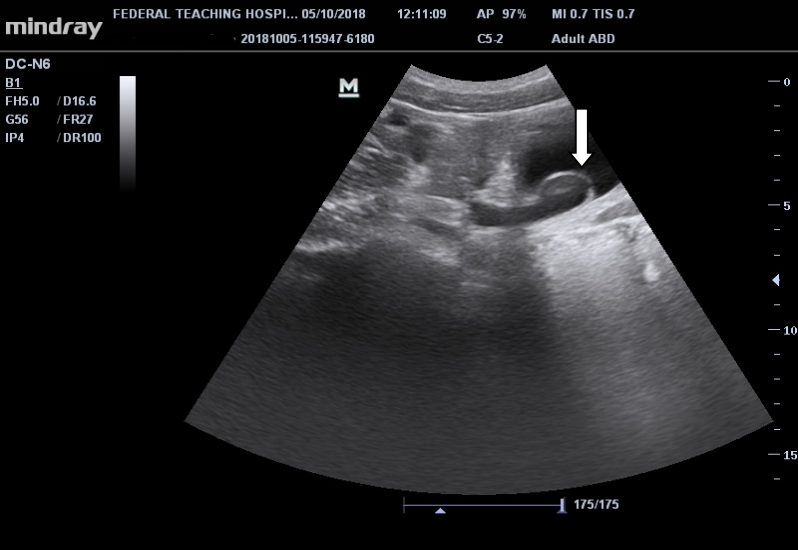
Ultrasonographic image of the urinary bladder showing dilated intravesical right ureter with cobra head sign (white arrow)
